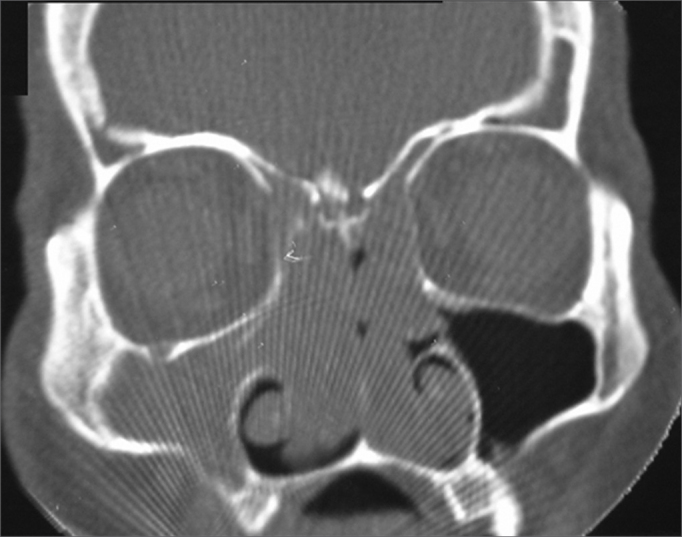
Nasal cavity and paranasal sinuses CT scan. Coronal cross-section, showing the bilateral involvement of nasal cavities, ethmoidal sinuses and right maxillary sinus.
